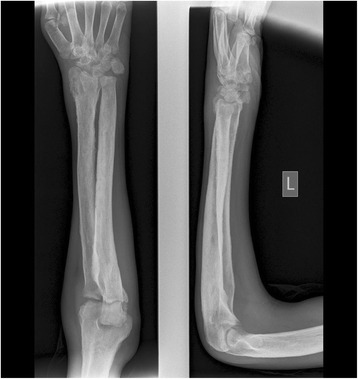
X-rays of ulna and radius showing periosteal thickening of both ulnae and medial radial shafts
